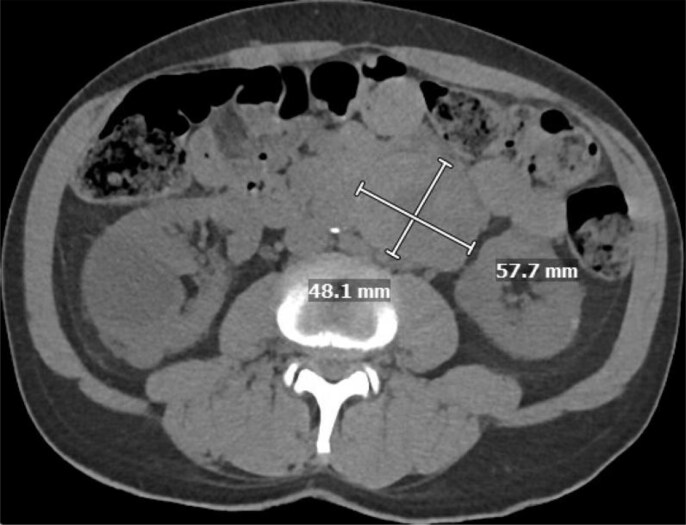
Computed tomography abdomen without contrast demonstrating para-aortic paraganglioma
